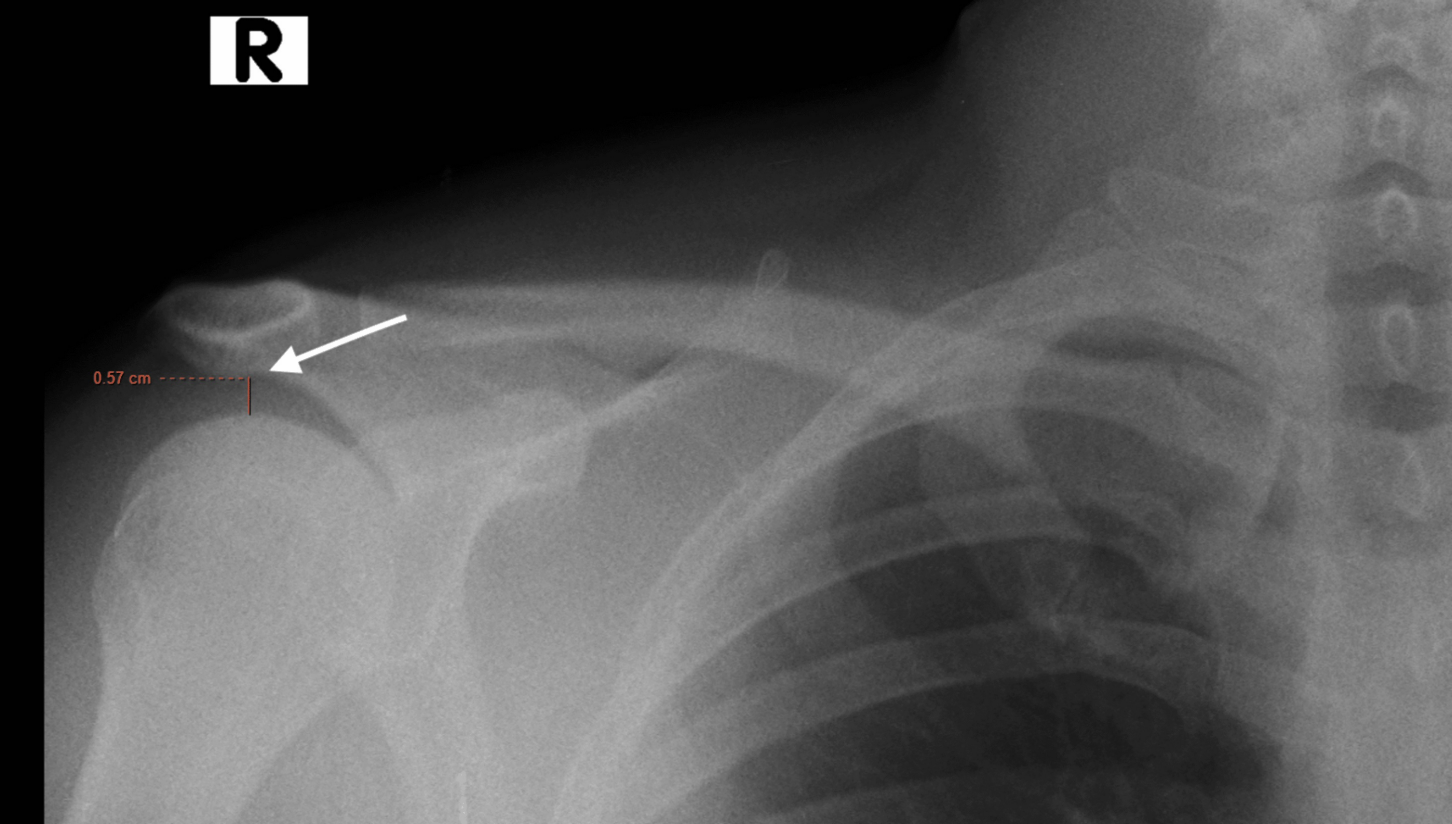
Postero-anterior (PA) view of the chest radiograph zoomed in image shows acromiohumeral index (AHI) - 0.57 mm on the right side.
